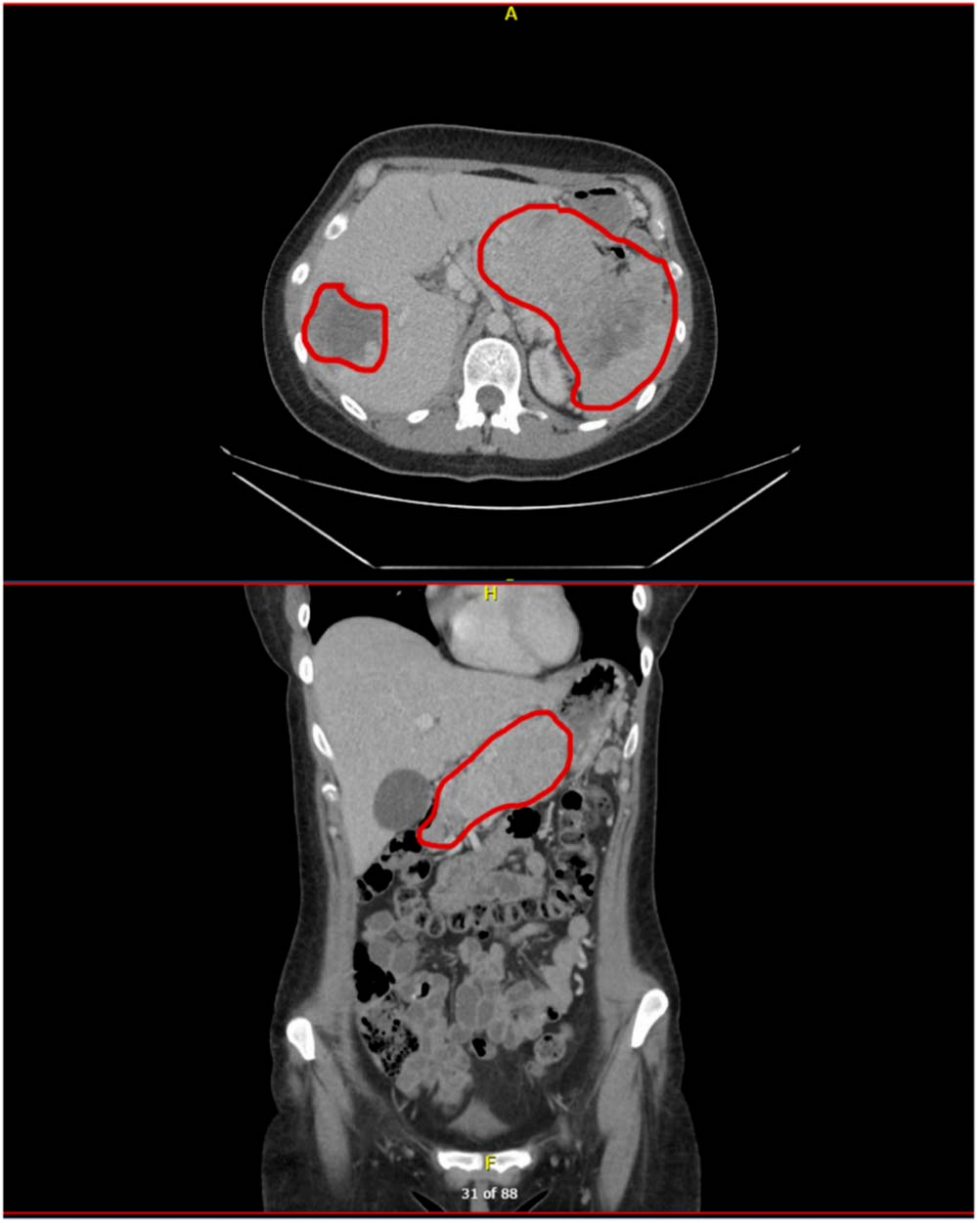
Case 2: contrast-enhanced axial (top) and coronal (bottom) CT images showing a large pancreatic mass, involving the body and tail, extending into the spleen and splenic flexure of the colon, with a large necrotic liver metastasis.
